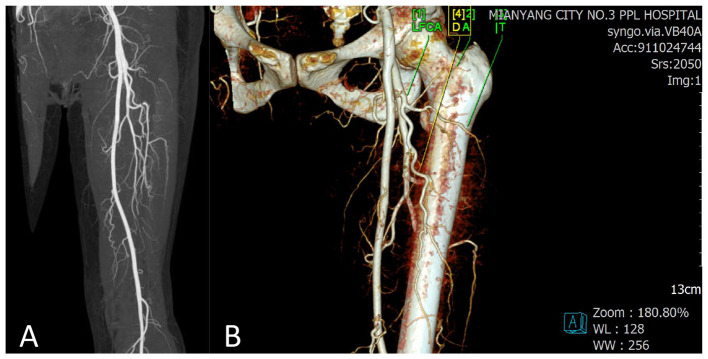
A, CTA image. B, Three-dimensional reconstruction of the perforator vessels of the anterolateral thigh flap.
